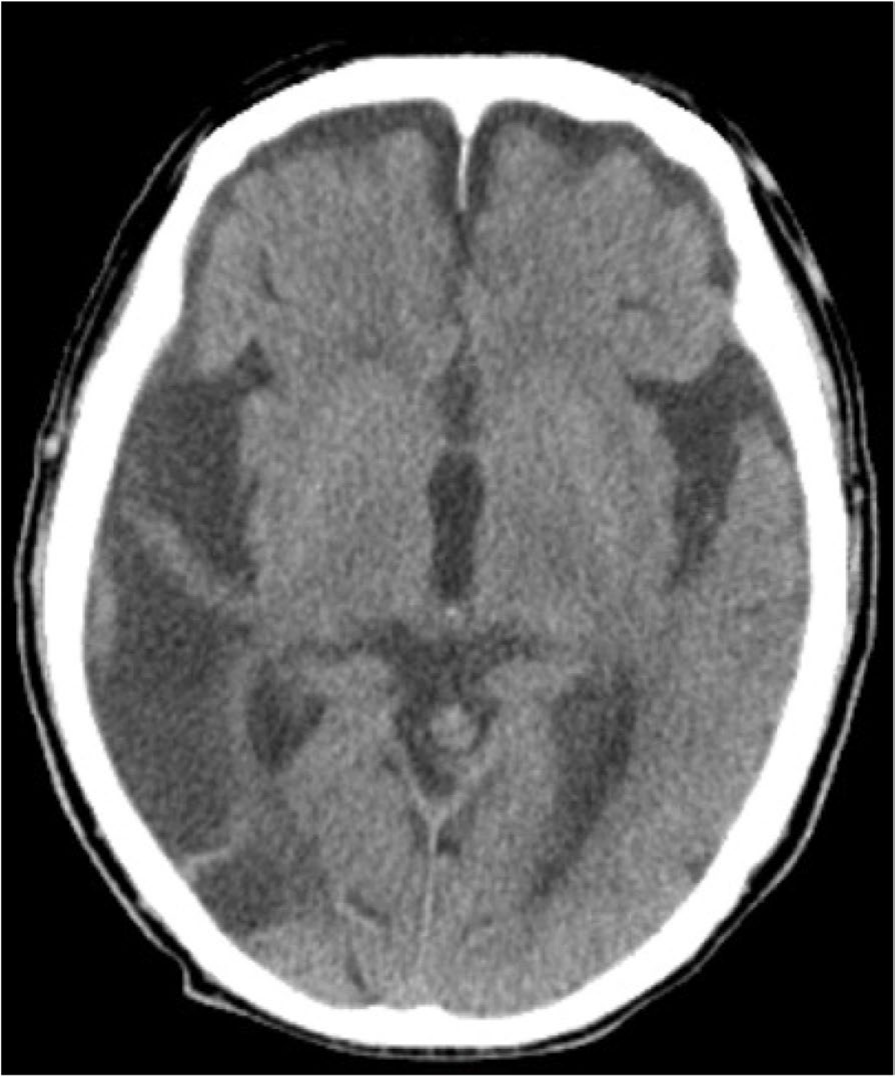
Computed tomography of the head. The arrow indicates an old cerebral infarction in the right middle cerebral artery region
